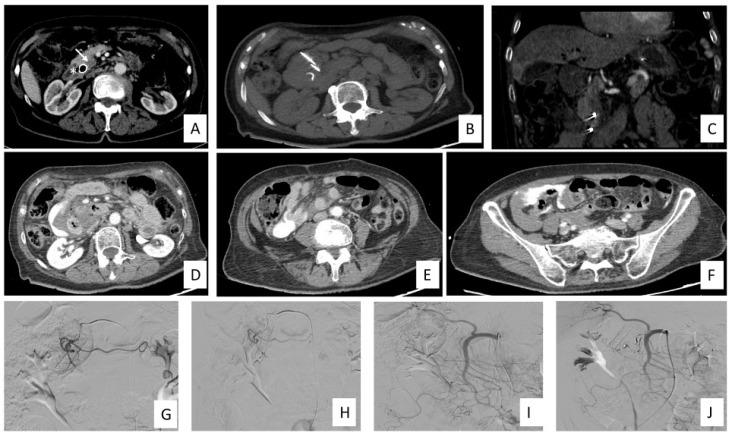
IRE for LAPC treatment timeline and complication management in a 76-year-old female patient. (A) CECT in arterial phase demonstrates the presence of the LAPC in the head of the pancreas (white arrows) and biliary stent (asterisk) prior to IRE treatment. (B) Axial view of noncontrast scan shows two needle electrodes in situ. (C) Coronal view in arterial phase shows the two electrodes in situ. (D–F) CECT scan immediately post procedure shows the presence of intraabdominal hematic fluid and extravasation of contrast media without any visible source of bleeding. (G–J) Angiography of celiac trunk, SMA, GDA, right renal artery and phlebography of the inferior vena cava and right renal vein did not demonstrate any source of bleeding. A preventive endovascular embolization of GDA and PDA were made using 3, 4 and 5 mm micro coils. Abbreviations: contrast-enhanced CT (CECT), irreversible electroporation (IRE), gastroduodenal artery (GDA), locally advanced pancreatic cancer (LAPC), pancreatic duodenal artery (PDA), superior mesenteric artery (SMA).
